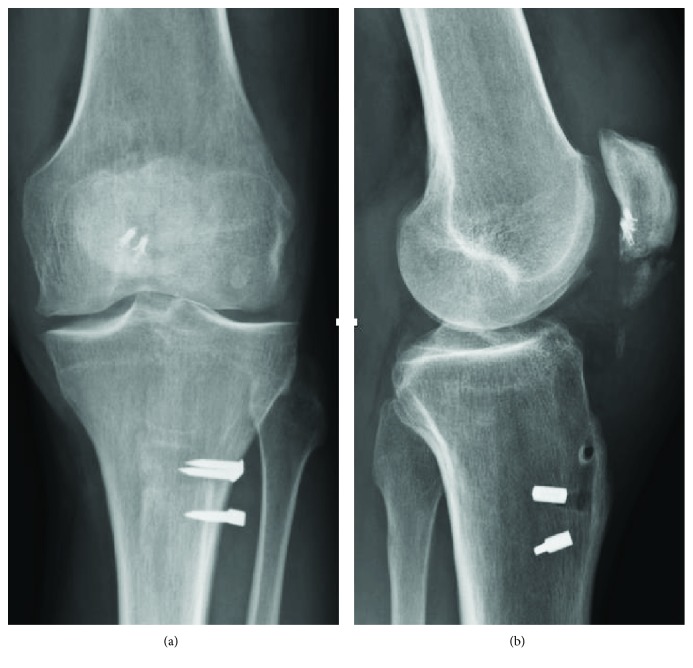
(a) Anteroposterior and (b) sagittal postoperative X-rays at 3 months.
